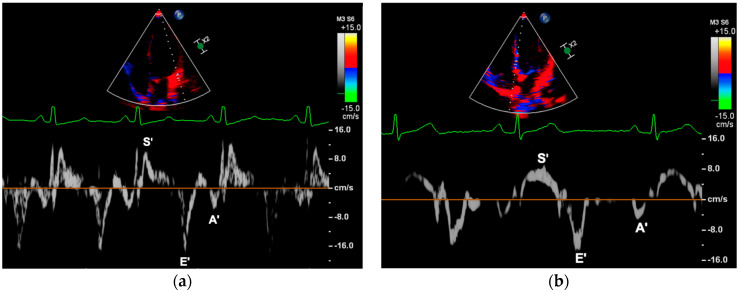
Measurement of myocardial peak velocities; (a) left ventricular wall, (b) interventricular septum. Images were obtained by placing the sample volume on the lateral and septal mitral annulus in an apical four-chamber view. Myocardial systolic (S’, cm/s), early diastolic (E’, cm/s), and late diastolic (A’, cm/s) peak velocities were measured offline.
